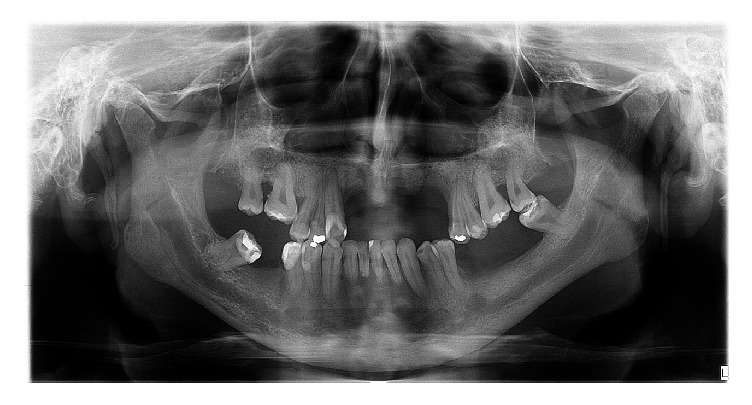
Panoramic Radiograph of the case. Enlargement of mandibular canal in both sides.
